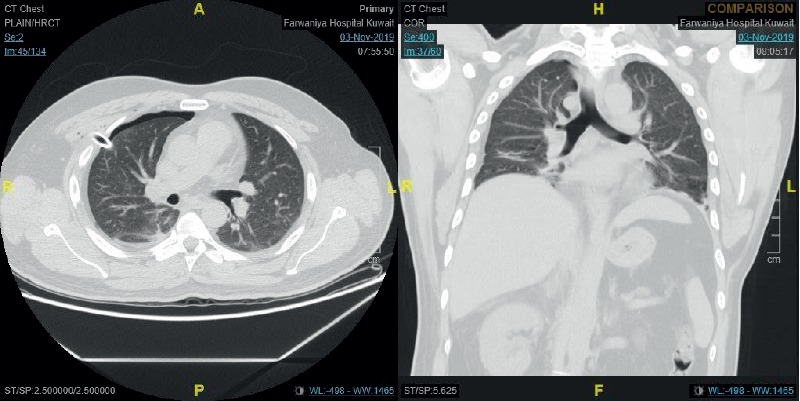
Postoperative computed tomography (CT) scan showing minimal right-side residual pneumothorax and the intercostal tube in place.
